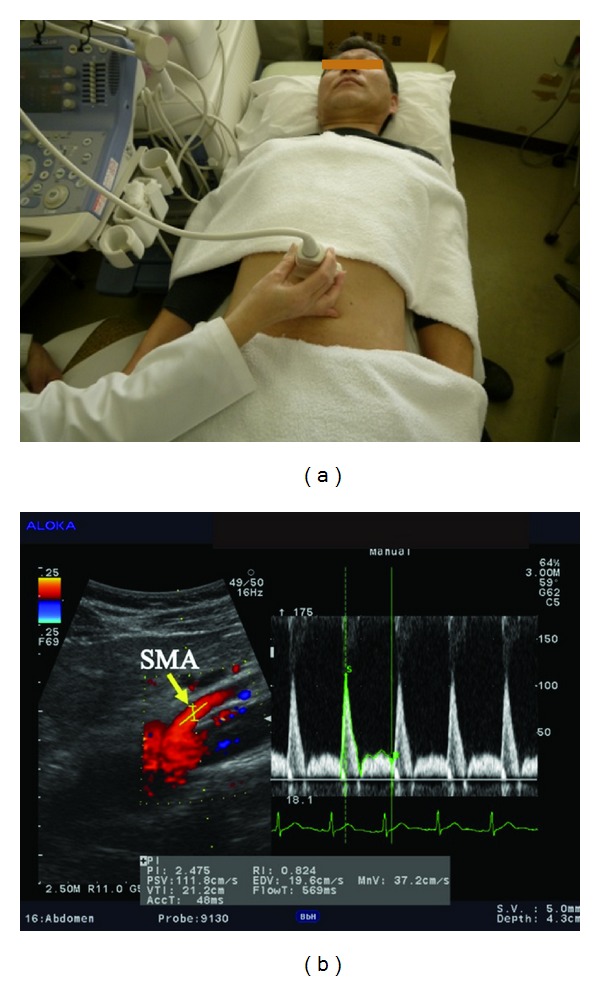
(a) Ultrasound measurement of the SMA. 5 MHz convex transducer is positioned on the abdomen. (b) Display of CDI. Left: image of the vessel and the position of the artery tracking. Right: Doppler flow and flow velocity.
